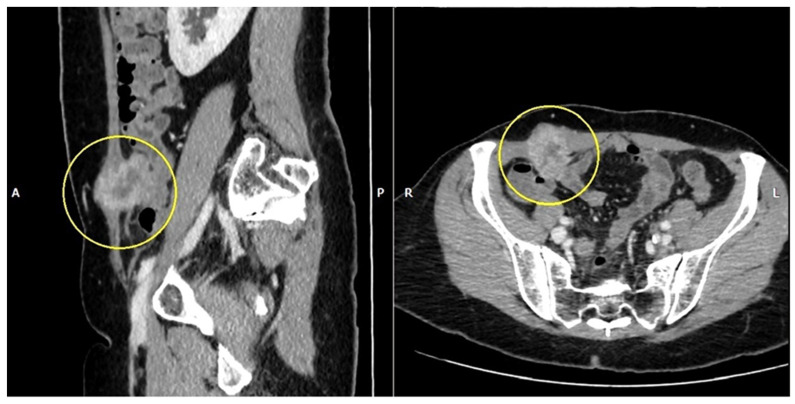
Abdomino-pelvin CT scan shows the parietal tumor in the right iliac fossa (yellow circles).
